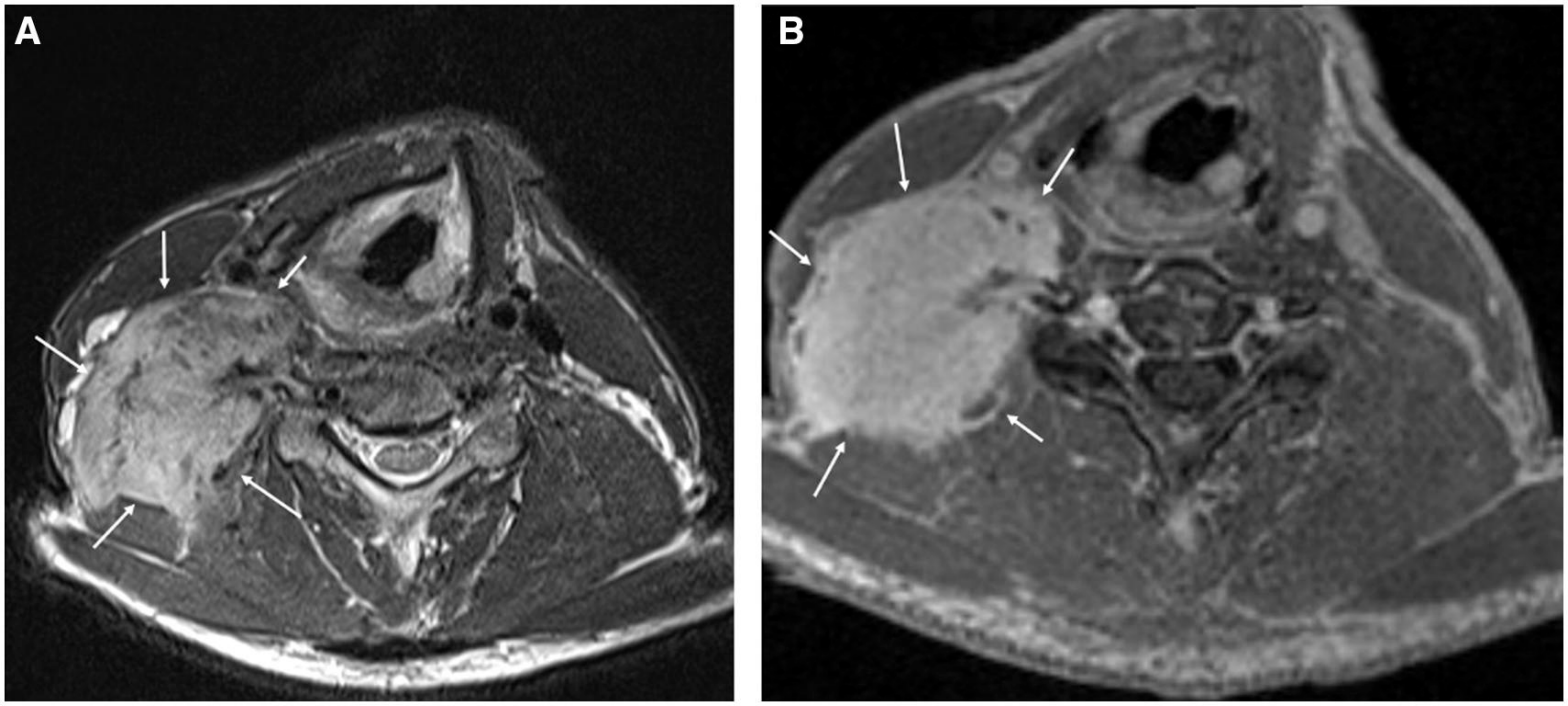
Desmoid fibromatosis. (A) Axial T2-weighted MRI shows an ill-defined lesion infiltrating the right scalene muscles and levator scapulae (solid white arrows). The tumour is predominantly T2-weighted hyperintense; intralesional heterogeneity reflects varying quantities of myofibroblasts, extracellular collagen, and myxoid matrix. Linear low-signal radiating bands correspond to collagen bundles. (B) Non-fat-suppressed post-contrast T1-weighted MRI shows avid tumour enhancement (solid white arrows).
